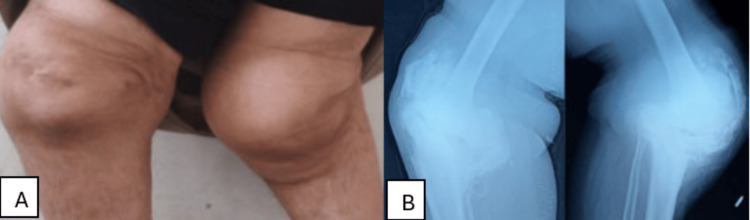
Imaging of Patient 3. (A) Clinical photograph of the knees demonstrating knee deformity. (B) Anteroposterior radiograph of both knees revealing bilateral destruction and malalignment.
